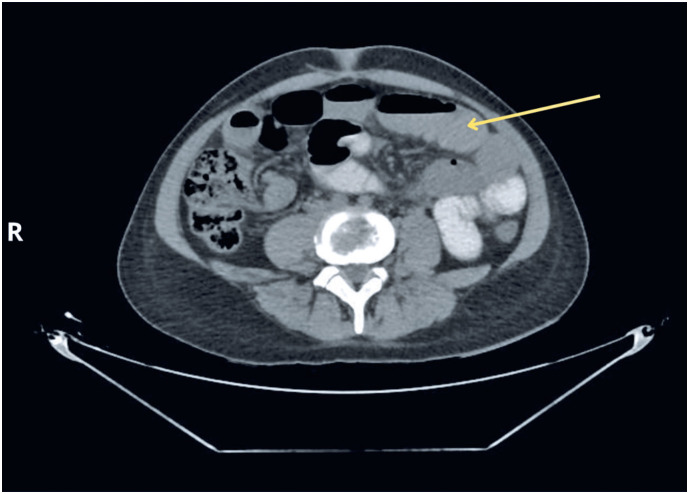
CT-Scan showing air-fluid level and small bowel dilatation
